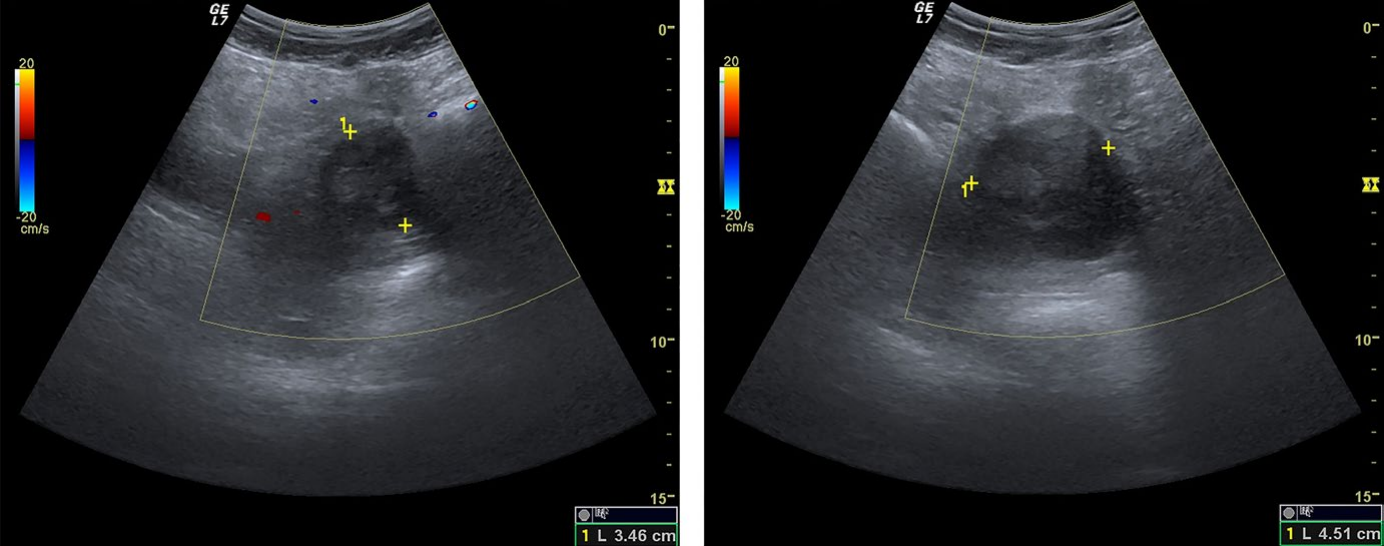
Abdominal ultrasound on 27 September 2014 shows a mass in the pelvis (4.51 × 3.46 cm)
